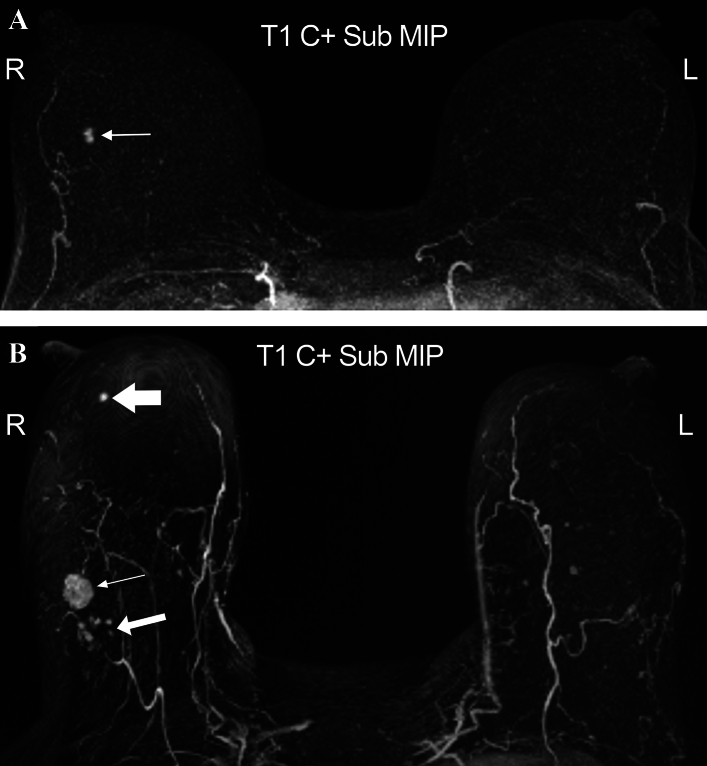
A 7 mm spiculated mass right breast at 11 o’clock. MRI shows minimal BPE and the unifocal index mass (arrow). Omission of RT on trial. B 15 mm spiculated mass right breast at 8 o’clock. MRI shows mild BPE and the index right mass (thin arrow) with adjacent occult NME (intermediate arrow). Additional ipsilateral MR-only mass at 8 o’clock (thick arrow). MR Bx of the additional ipsilateral mass = IC NST. Exclusion from omission of RT
